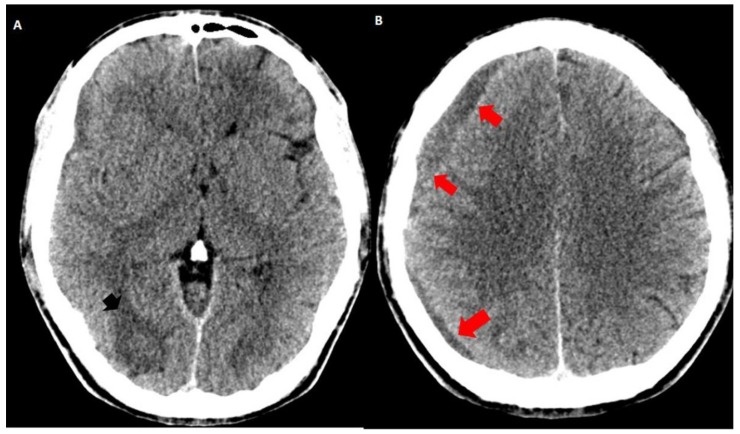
Simple brain CT one month later: the increased hypodensity of the intraparenchymal (black arrowhead in (A)) and subdural hematomas (red arrows in (A,B)) indicate hemoglobin degradation. The frontal subdural hematoma slightly increased its volume and extended to the right parietal region (red arrows).
